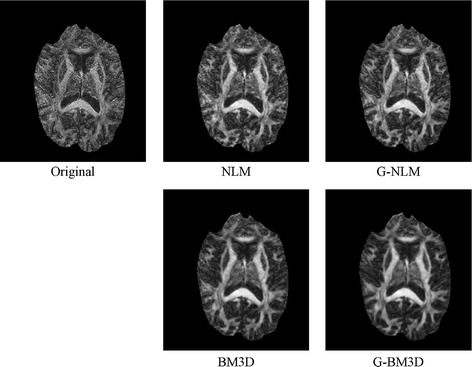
Denoising results for the FA map of the DWI dataset
